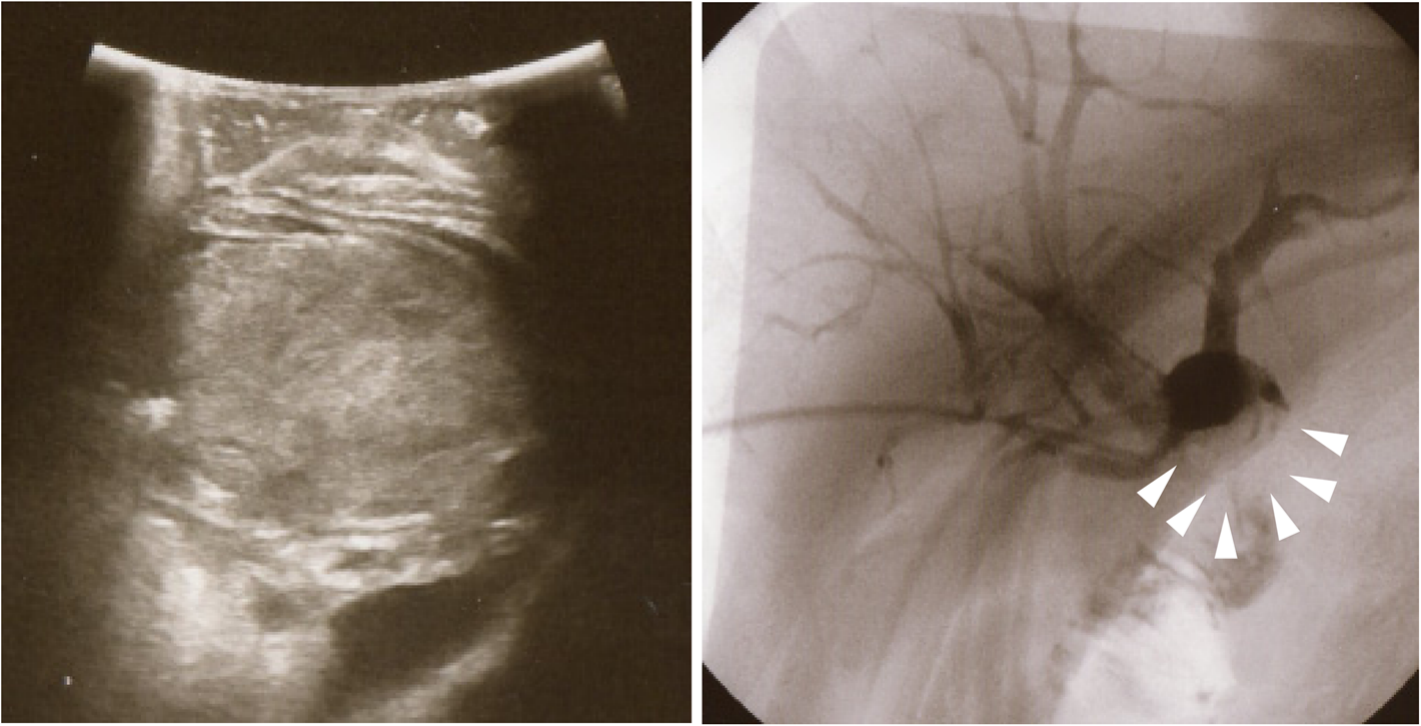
Ultrasonography showed intrabiliary growth (*left panel*) and percutaneous transhepatic cholangiography showed filling defect in the choledocojejunostomy (*arrows in right panel*)
